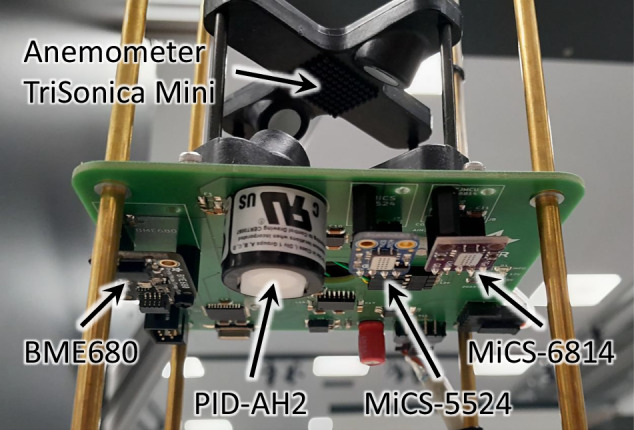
Photo of one individual sensor platform. The different gas and PHT sensors were mounted on the underside. The ultrasonic anemometer was installed on top.
